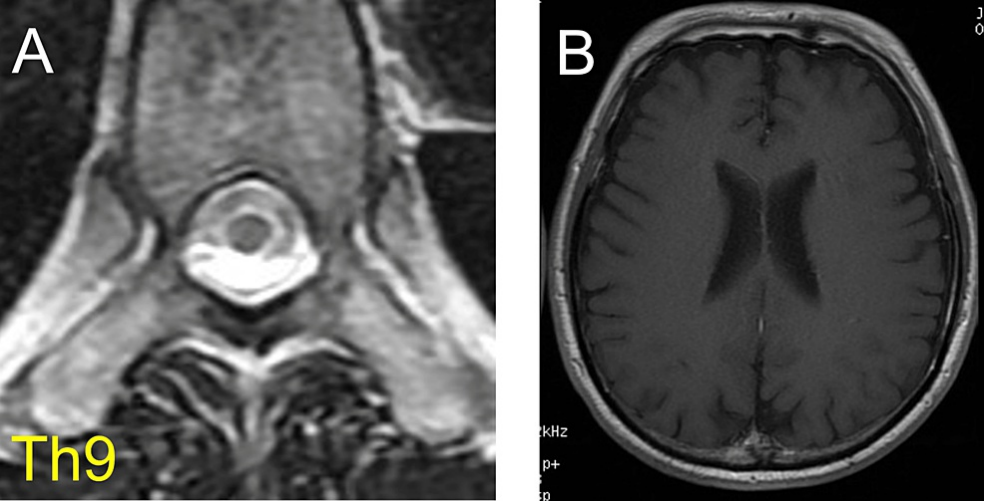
Spinal and brain MRI findings in patient 2 (A) A spinal MRI shows the atrophy of the thoracic cord on the ninth thoracic vertebra level. (B) On brain MRI, contrast-enhanced T1-weighted imaging does not show enhanced lesions and atrophy
